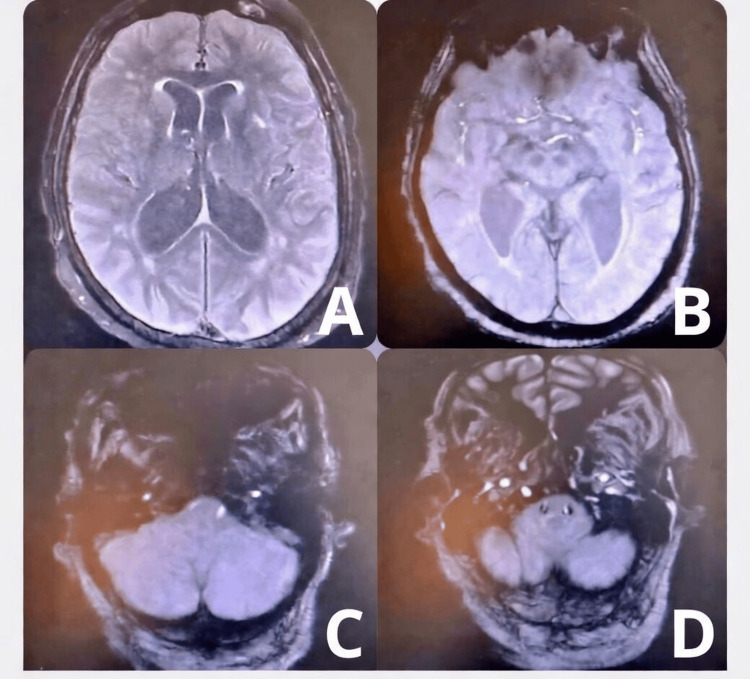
Brain MRI, axial slices (A) High-supratentorial T1 sequence, lateral ventricles symmetrical with preserved morphology; (B) Mid-supratentorial T2-weighted Fluid-Attenuated Inversion Recovery (T2/FLAIR) sequence, symmetrical deep structures and median third ventricle not dilated; (C) Low-supratentorial T2 sequence, apparent integrity of cerebral parenchyma; (D) Low-supratentorial T2 sequence, cerebellum with preserved morphology, symmetrical, visible fourth ventricle not compressed and brainstem with regular appearance. No abnormalities, particularly of the brainstem, explaining the symptoms.
